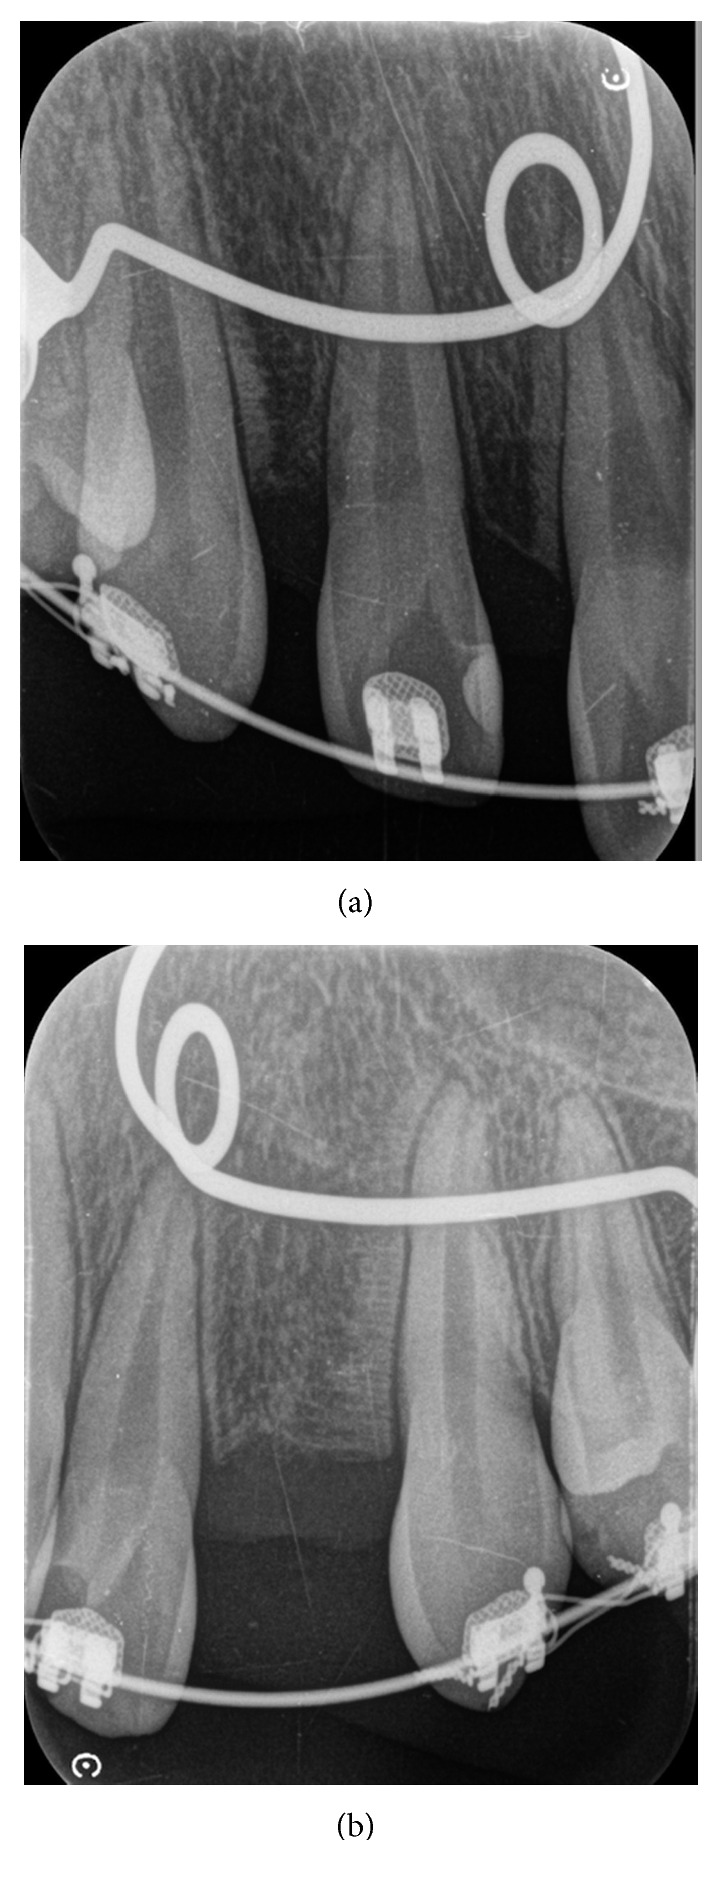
Periapical radiographs at T1. (a) Control side; (b) MOP side.
